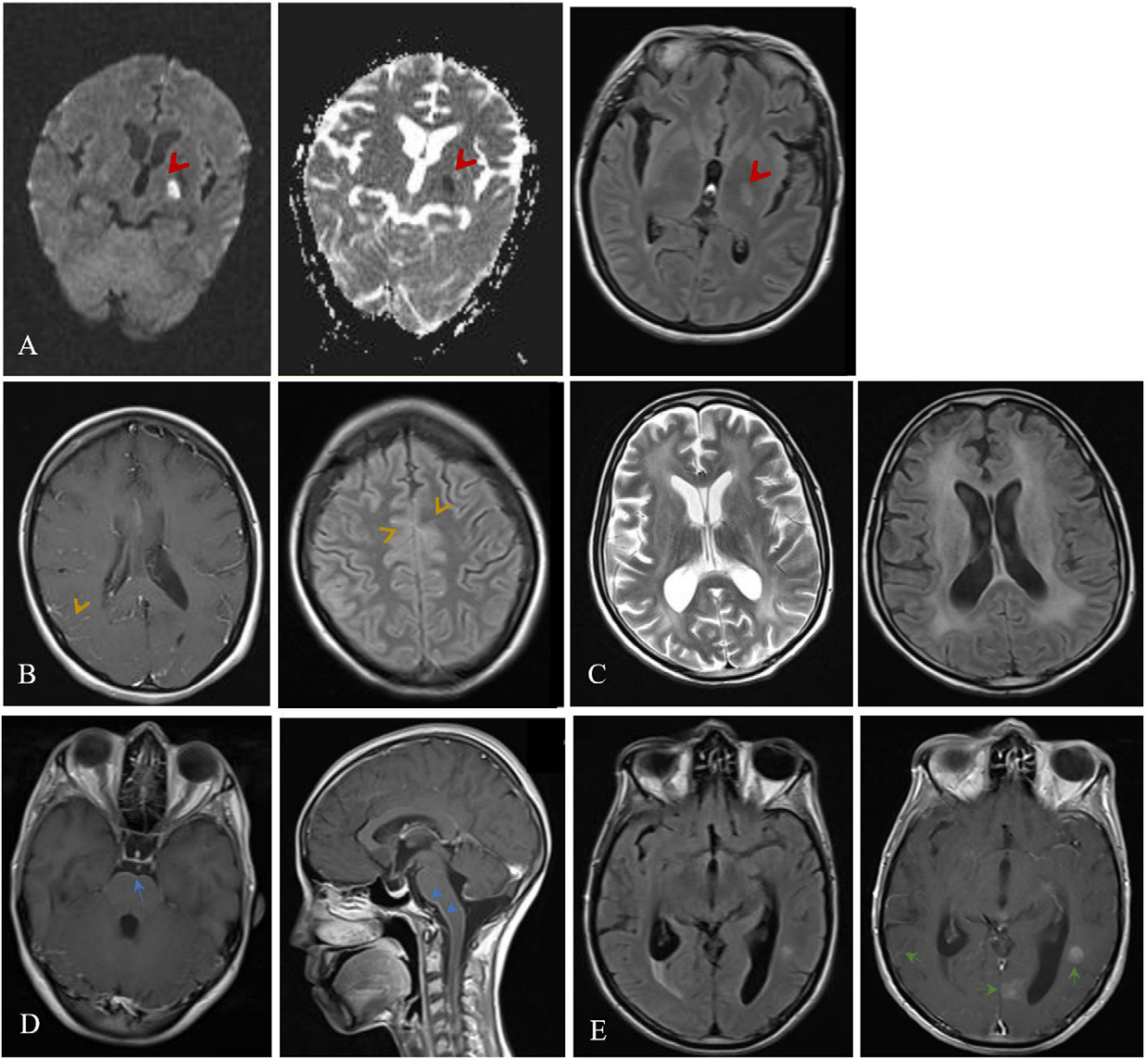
Abnormal neuroimaging findings in patients with subacute and chronic meningitis. (a) A 33-year-old woman with CNS tuberculosis complicated with brain infarct. Diffusion-weighted image (DWI) and ADC show restricted diffusion in left basal ganglia, hallmark feature of acute infarction (left images) and FLAIR image shows high signal intensity in left basal ganglia corresponding to the lesion seen on DWI and ADC. (b) A 19-year-old woman with CNS tuberculosis diagnosed early after a stillbirth. Post contrast T1 weighted image shows leptomeningeal enhancement and FLAIR image demonstrates sulcal hyperintensity (CSF dirty sign). (c) A 36-year-old man, new case of HIV infection with cryptococcal meningoencephalitis and HIV-associated leukoencephalopathy who died before antifungal treatment was started. T2 weighted and FLAIR images show hydrocephalus and white matter hyperintensity. (d) A 27-year-old woman with Brucella meningitis presented with multiple cranial nerve palsy. Post contrast T1 weighted images show leptomeningeal enhancement around brain stem. (e) A 46-year-old man with carcinomatous meningoencephalitis presented with multiple cranial nerve palsy. Post contrast T1 weighted image demonstrates leptomeningeal enhancement and multiple enhancing lesions.
